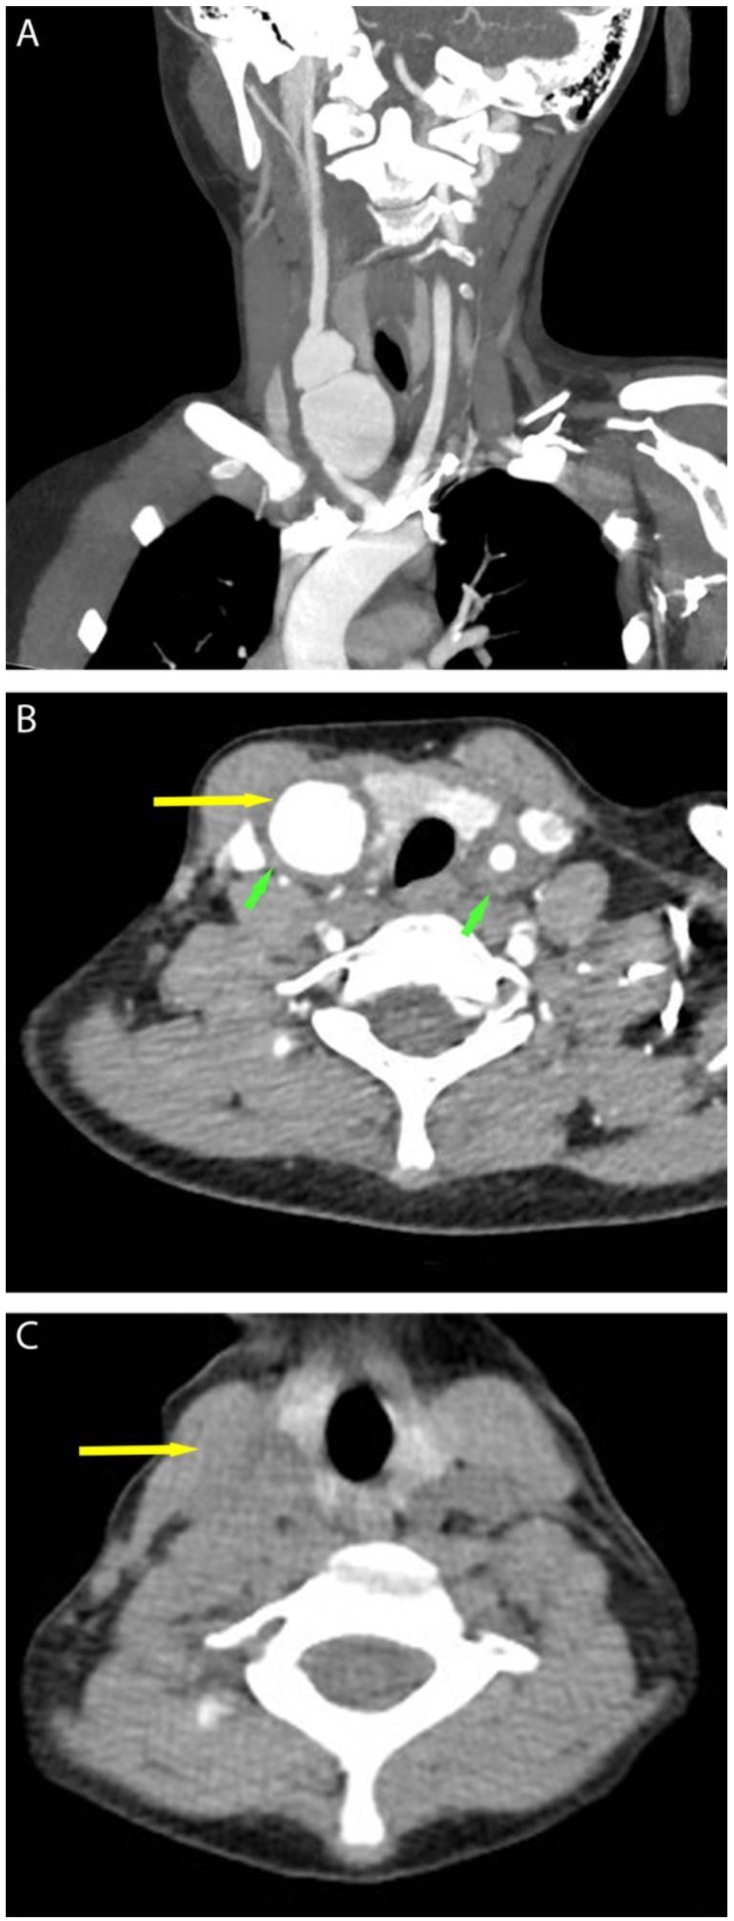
(A) Coronal plane of CT angiography showing dilation of the carotid arteries. (B) Transverse plane of CT angiography showing dilation (yellow arrow) and wall thickening (green arrows) of carotid arteries. (C) Chest CT before antimony treatment showing a pre-existing soft-tissue mass at the right common carotid artery region (arrow).
